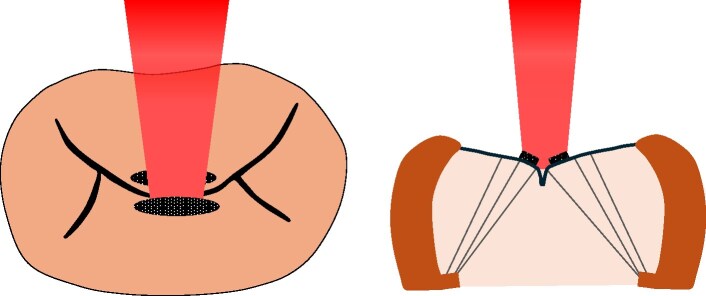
Echocardiography demonstrated severe mitral regurgitation (MR) with non-bacterial thromboendocarditis (NBTE)
